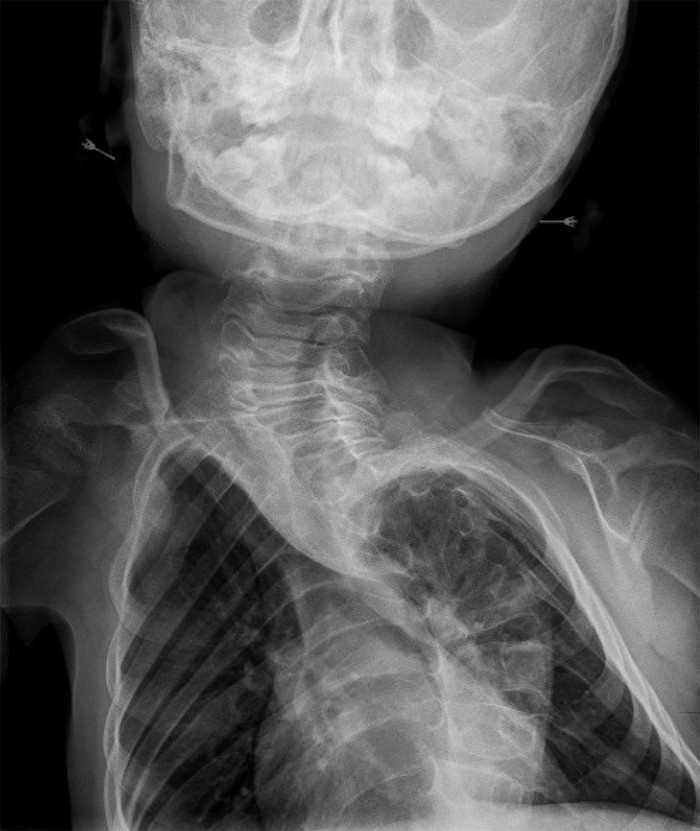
Radiograph of cervical and thoracic spine of the female sibling at age 5 showing severe spinal deformity and autofusion.
